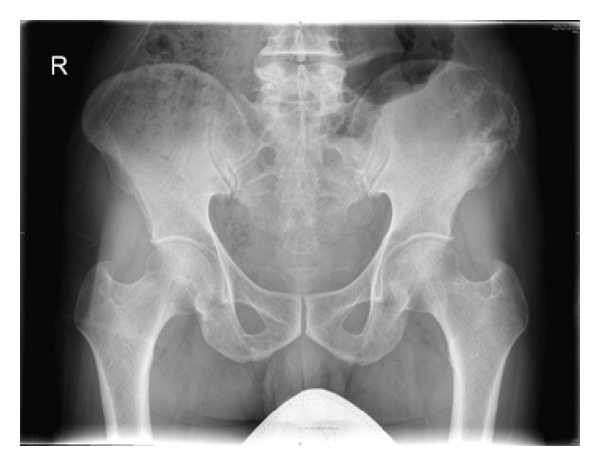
Plain radiograph of Pelvis showing an osteolytic lesion with indistinct margins, cortical erosion, and narrow transitional zone over the left ilium.
